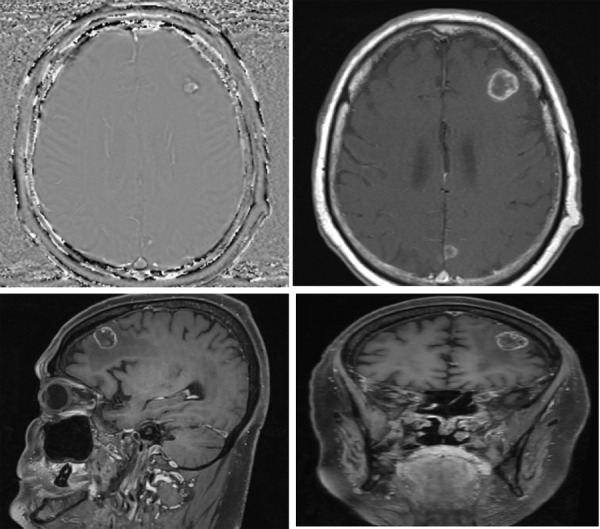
CT scan of the head shows brain metastases anteriorly in the left frontal lobe and in the central occipital lobe, prior to chemotherapy.
